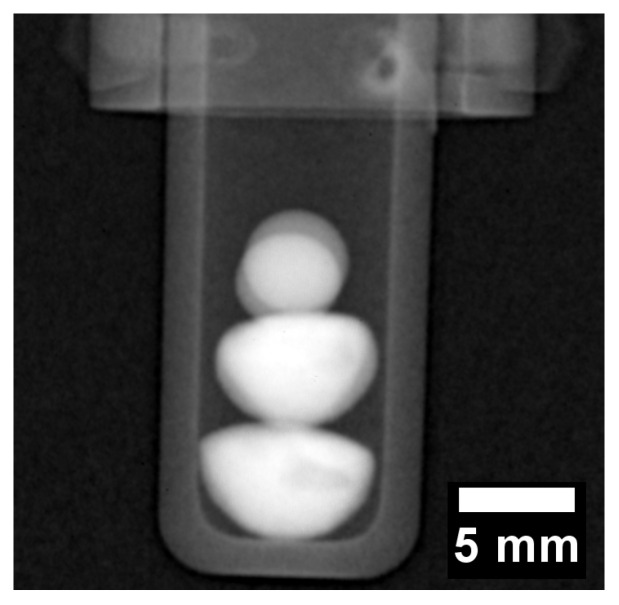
Radiograph of the four buttons stacked inside an alumina crucible such that the heterogeneous CoCrCu are placed on the bottom while the spheres of Ni are placed at the top (the Ni buttons are oriented in the direction of the beam such that they overlap). The darker regions present randomly in the bottom two buttons are the Cu-rich phase that separated in the liquid during arc-melting of the CoCrCu buttons.
